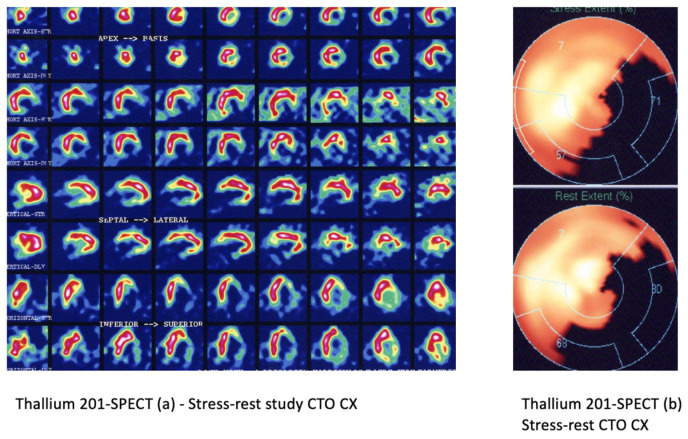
Thallium 201-SPECT study in a patient with a CTO CX as described in the coronary angiography. Short axis views are displayed in row 1–4 and vertical and horizontal long axis views in row 5–8. No significant tracer uptake in stress (row 1,3,5,7) and rest (row 2,4,6,8) acquisitions can be interpreted as lack of viability in the lateral and basal part of inferior myocardial segments (a). On the right, a semi-quantification of tracer uptake from the stress (top) and rest (bottom) acquisitions in a polar map (b).
